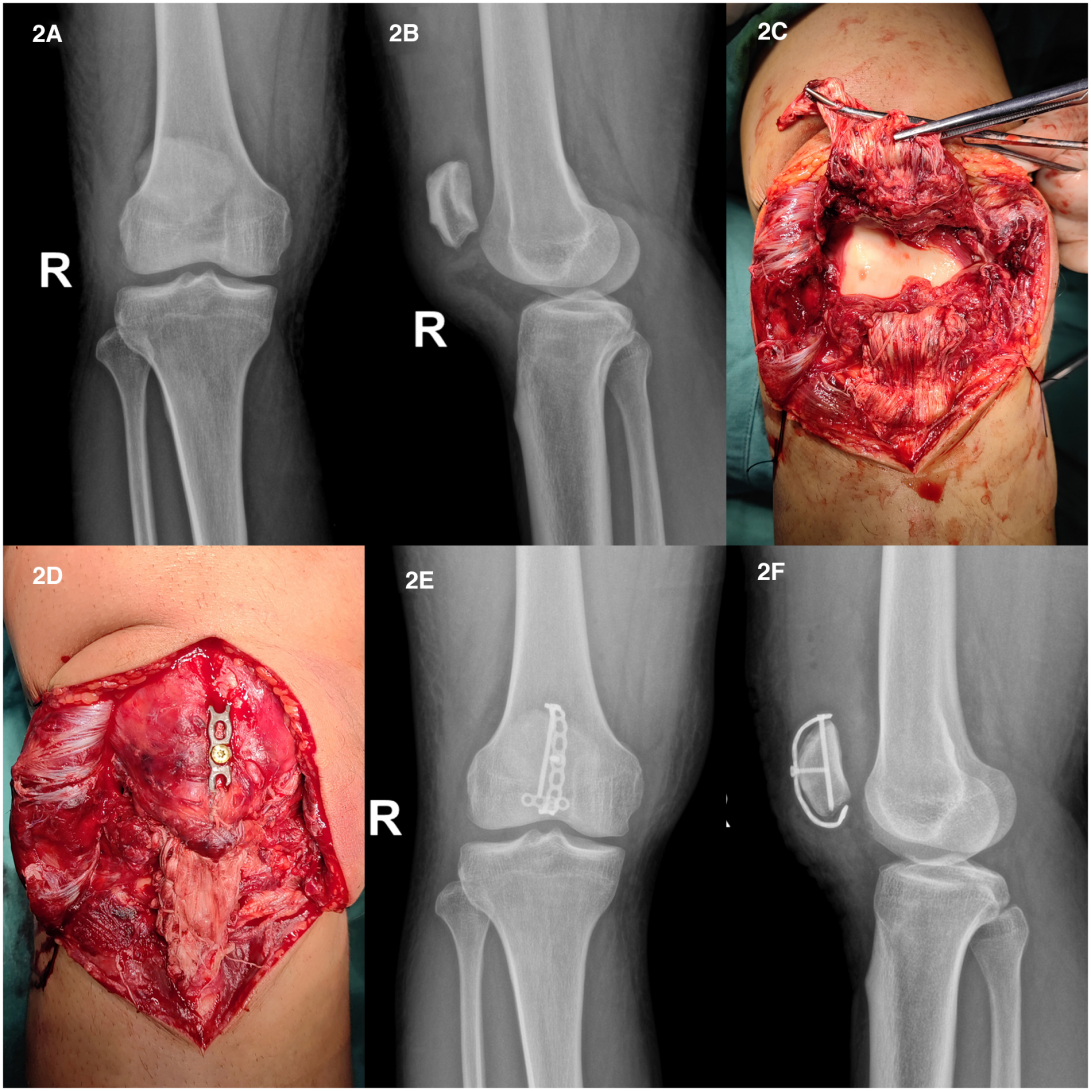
(A,B) The anterior and lateral x-ray film of knee joint; (C) complete rupture of patellar tendon; (D) after the continuity of patellar tendon was reconstructed, the fixation was completed; (E,F) the anterior and lateral x-ray films of knee joint after operation.
